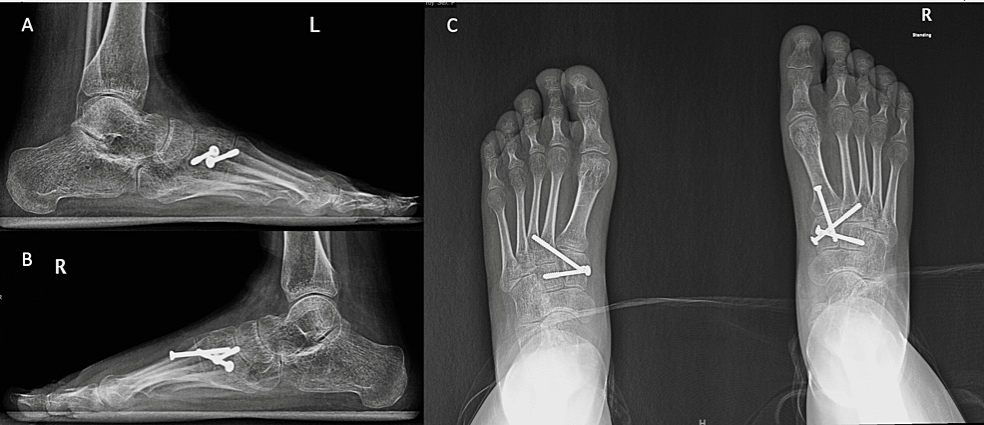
Weight-bearing anteroposterior and lateral x-ray images of both feet at postoperative six months (A) Weight-bearing left foot AP x-ray. (B) Weight-bearing left foot lateral x-ray. (C) Bilateral weight-bearing foot AP x-ray. AP: Anteroposterior.
